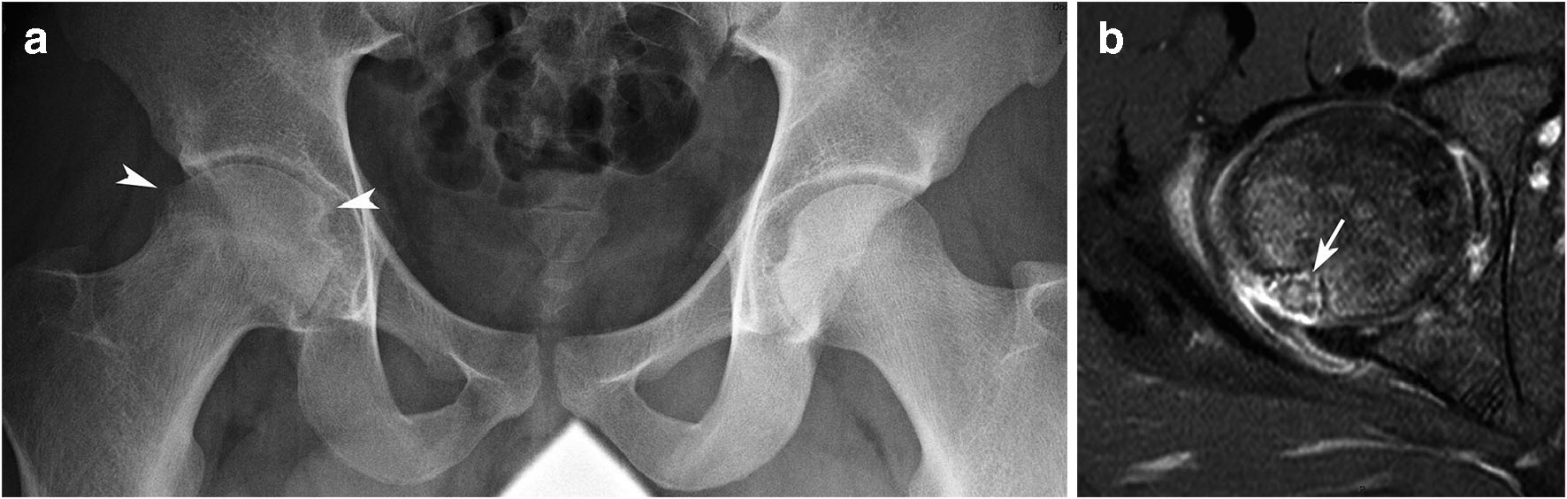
Femoral head OO mimicker of hip arthritis in a 19-year-old man with continuous right hip pain for 2 years despite drilling of the femoral head and an open synovial biopsy. a Pelvic radiograph demonstrates right hip osteoporosis with joint space narrowing and osteophytes (arrowheads) suggestive of chronic arthritis. Axial fat-saturated post-contrast T1-weighted MR image (b) demonstrated an OO in the posterior aspect of the femoral head (arrow)
